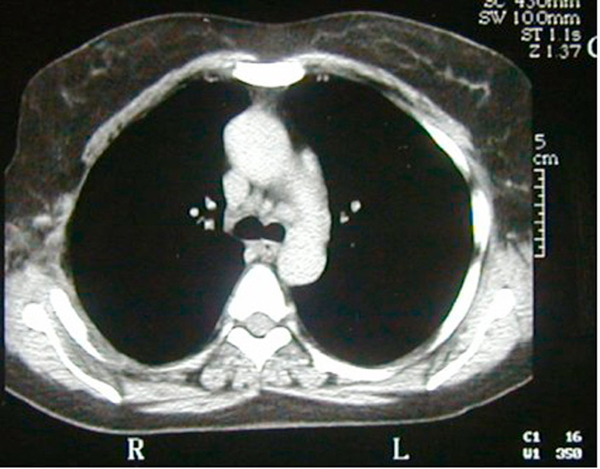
Computed tomography scan of the patient 5 years after the surgery with no evidence of recurrence.
